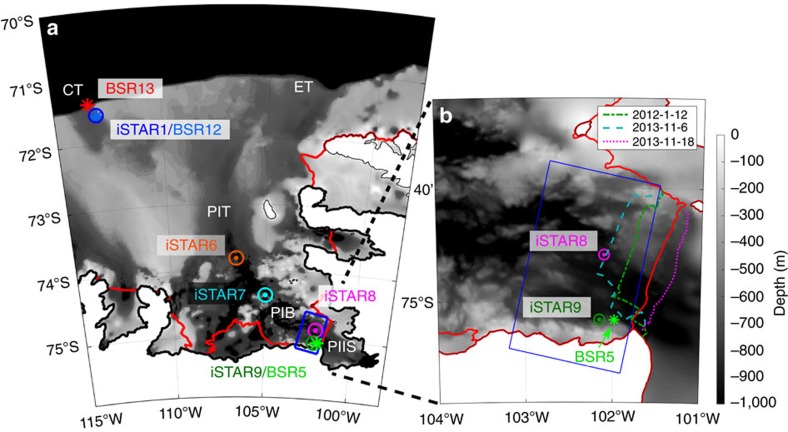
Ocean observations in the Amundsen Sea. (a) Location of study area; IBCSO bathymetry^41^ (shaded, see legend) and ice shelf edges from 2004 (red outlines); central (CT), eastern (ET) and Pine Island (PIT) troughs, Pine Island Bay (PIB), Pine Island Ice Shelf (PIIS); mooring locations (labelled; iSTAR moorings circles; BSR moorings stars) and the region over which the surface fluxes are averaged (blue box). (b) Enlargement of the Pine Island ice front showing the position of the ice front on 12 January 2012 (green dot-dash line), 6 November 2013 (before iceberg calved; cyan dashed line), 18 November 2013 (after iceberg calved; magenta dotted line).
